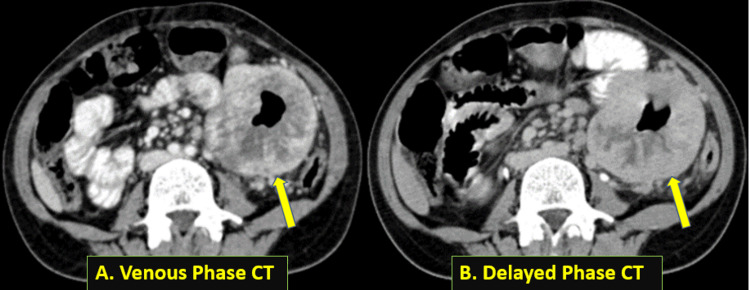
(A) An axial section of a venous phase-contrast enhanced computed tomography image (soft tissue window) demonstrating centripetal filling of the mass lesion with contrast (depicted by an arrow). (B) An axial section of a delayed phase-contrast enhanced computed tomography image (soft tissue window) demonstrating contrast filling of almost the entire lesion (shown by the arrow), with a few non-enhancing areas and central air collection.
